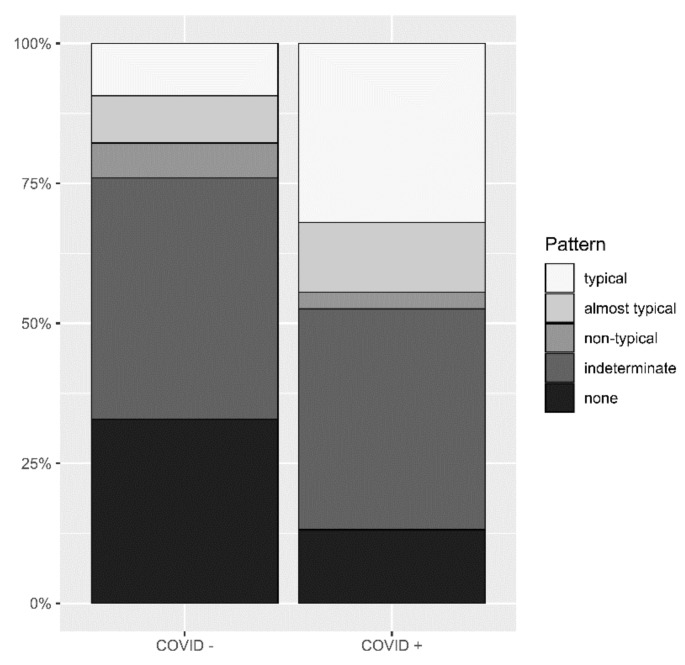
Different distribution patterns in patients with and without COVID-19. Significantly different distribution patterns of opacifications and/or consolidations in chest radiographs between patients with confirmed and ruled-out COVID-19 infections.
